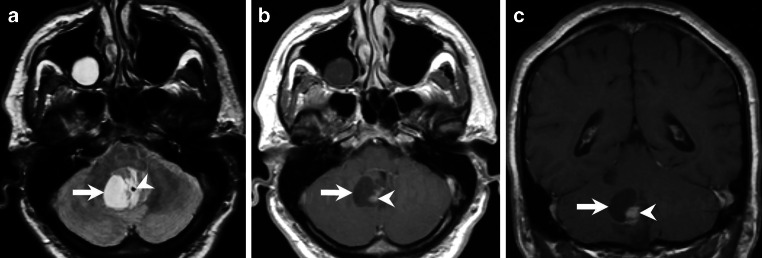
Preoperative magnetic resonance imaging (MRI) from 2009. Axial FLAIR (Fluid Attenuated Inversion Recovery) images (a) and coronal T2-weighted images (b) reveal a posterior fossa tumour with characteristic features of a pilocytic astrocytoma (a,b, *arrowhead*). On T1 weighted images obtained following intravenous administration of gadolinium (Gd) the solid component of the lesion showed homogeneous contrast enhancement (c, *arrowhead*). The solid tumour was surrounded by a large cystic portion (a–c, *arrow*). Neuropathological examination confirmed the diagnosis of pilocytic astrocytoma after microsurgical removal of the tumour in 2009
